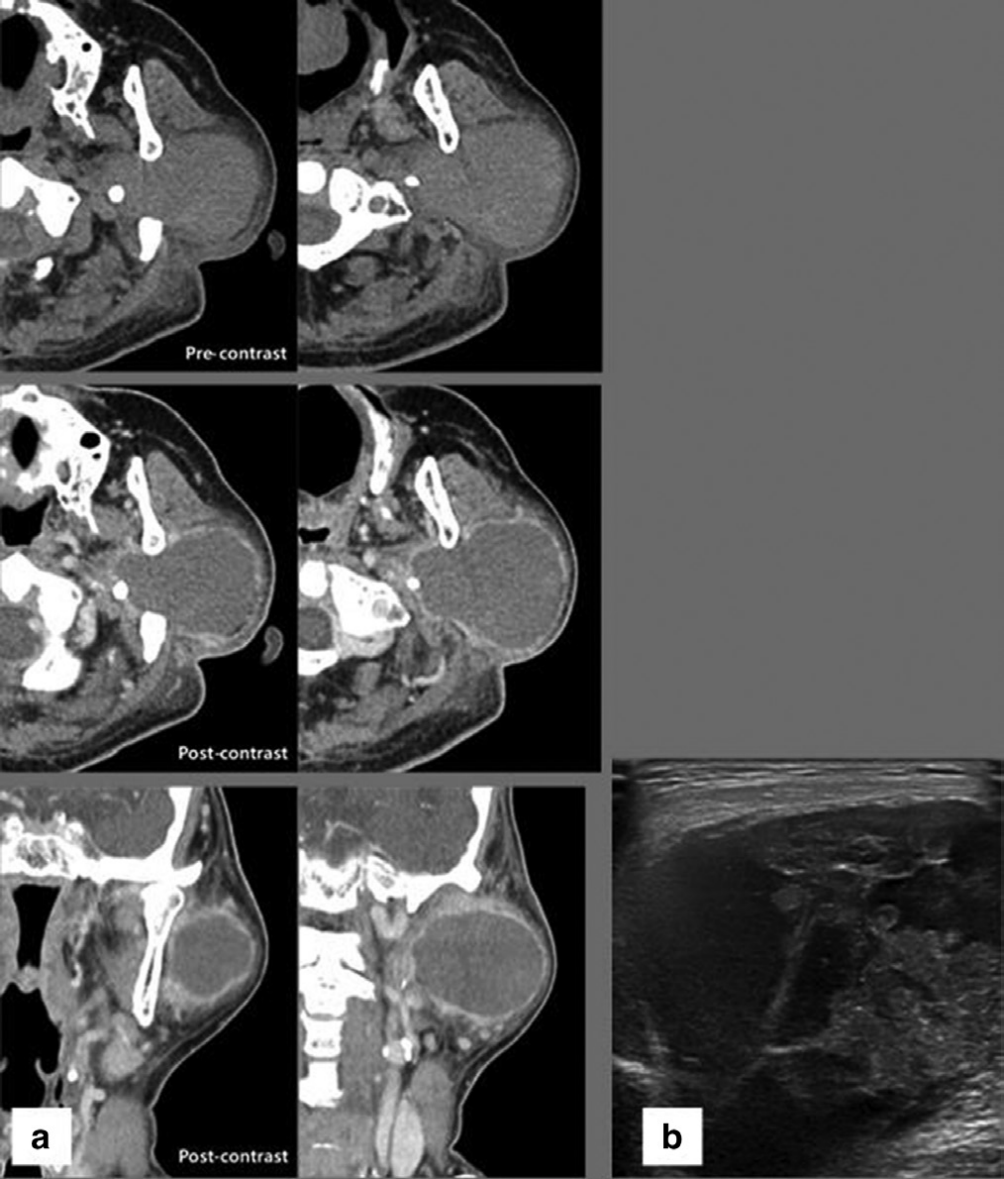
The same patient as Figure 1 with rapidly growing tender mass in the left preauricular and submandibular areas, after 8 years from initial imaging studies. (a) Pre-contrast axial CT scan reveals approximately 7 cm-sized homogeneously attenuated mass with perilesional fat infiltrations in the left parotid gland. The attenuation of its internal low density portion is measured as 47 HU. Contrast enhanced axial and coronal images reveal non-enhancing internal low density portion and peripheral thick enhancing rim of the mass. There is no definite enhancing nodular portion in the mass. (b) Sonogram shows large solid and cystic mass in both superficial and deep lobes of the left parotid gland. HU, Hounsfiled unit.
